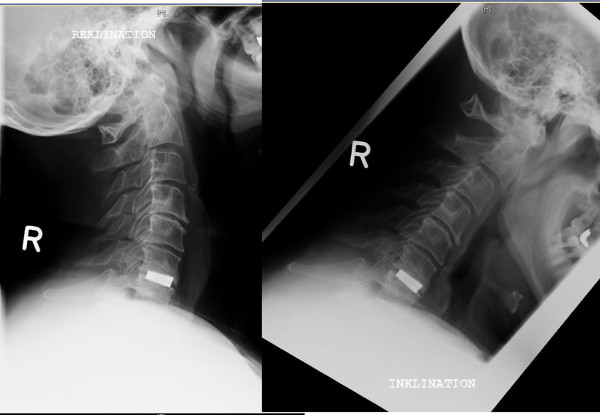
55-years old patient with a pseudarthrosis 3 years after surgery. The motion of the operated segment can be clearly seen by observing the movement of the spinous processes in the lateral functional x-rays.
